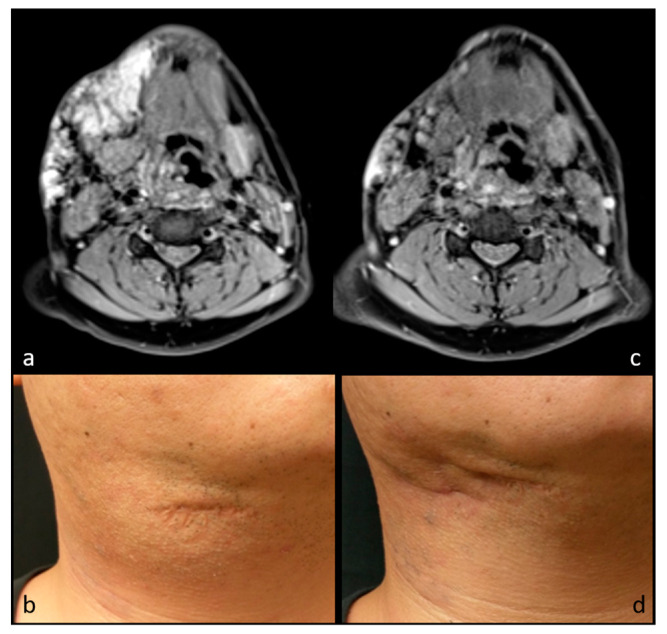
MR images and photographs of patient 3 before (a,b) and after (c,d) RFA (cosmetic scale 3 → 1). Previous surgical scar is also visible in photographs.
